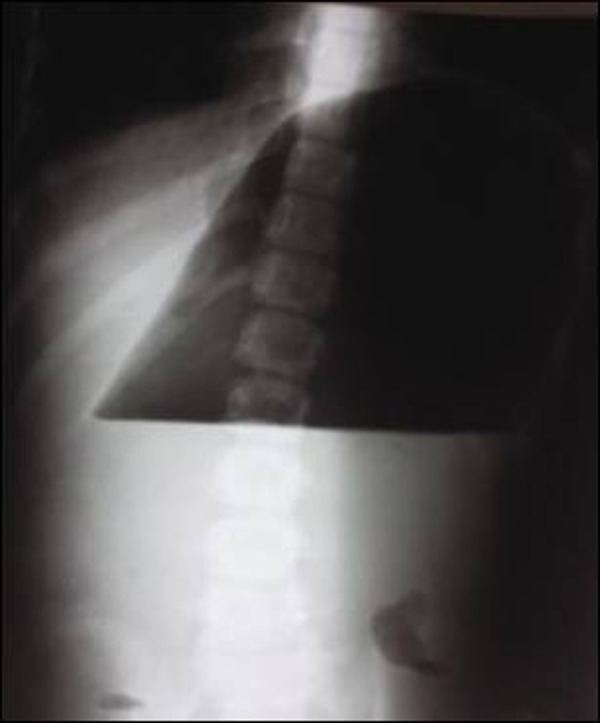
Figure 1: X-ray abdomen showing single air fluid level.
